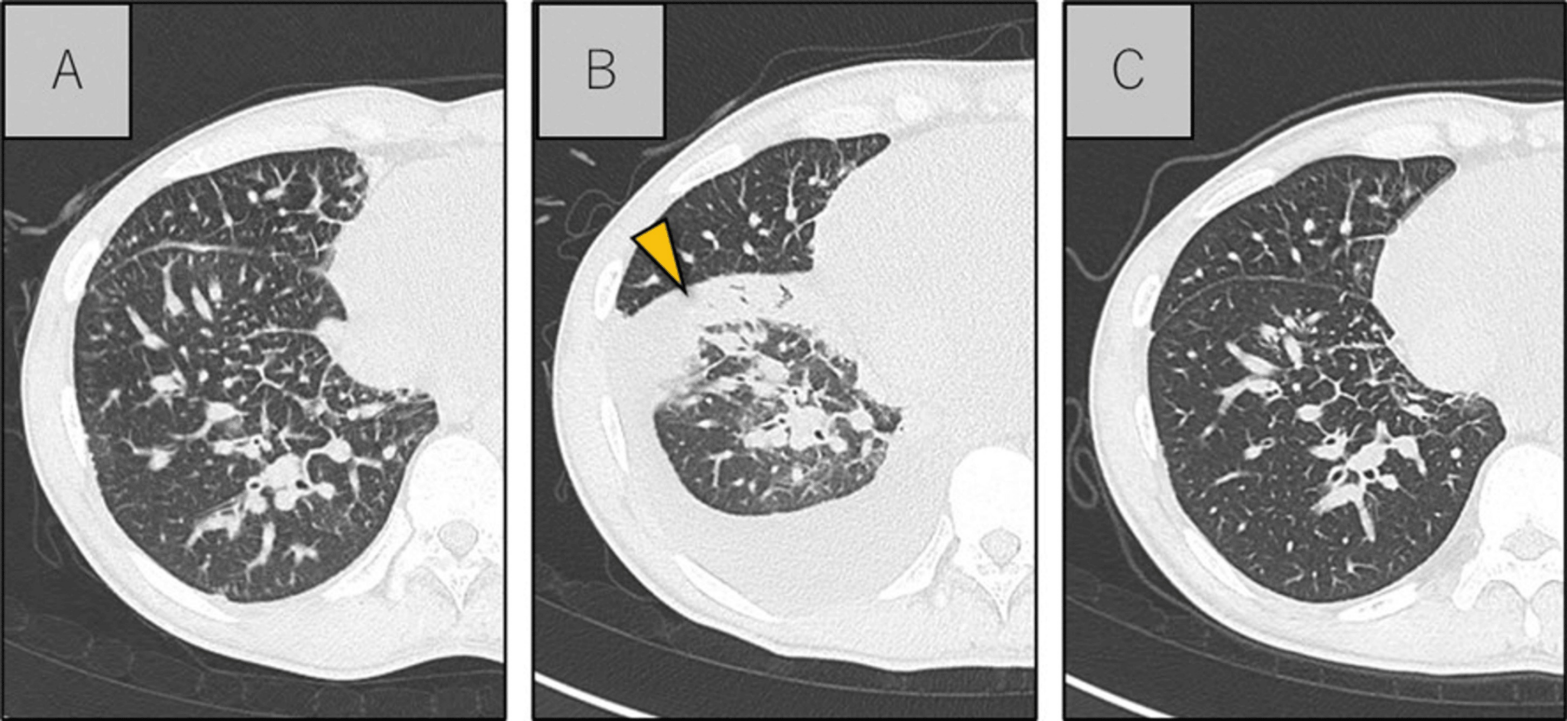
Progress shown on CT imaging A: Before starting crizotinib. B: On day 10 after the start of administration, pneumonia appears (yellow arrow). C: Pneumonia improves five days after the discontinuation of crizotinib. CT: computed tomography
